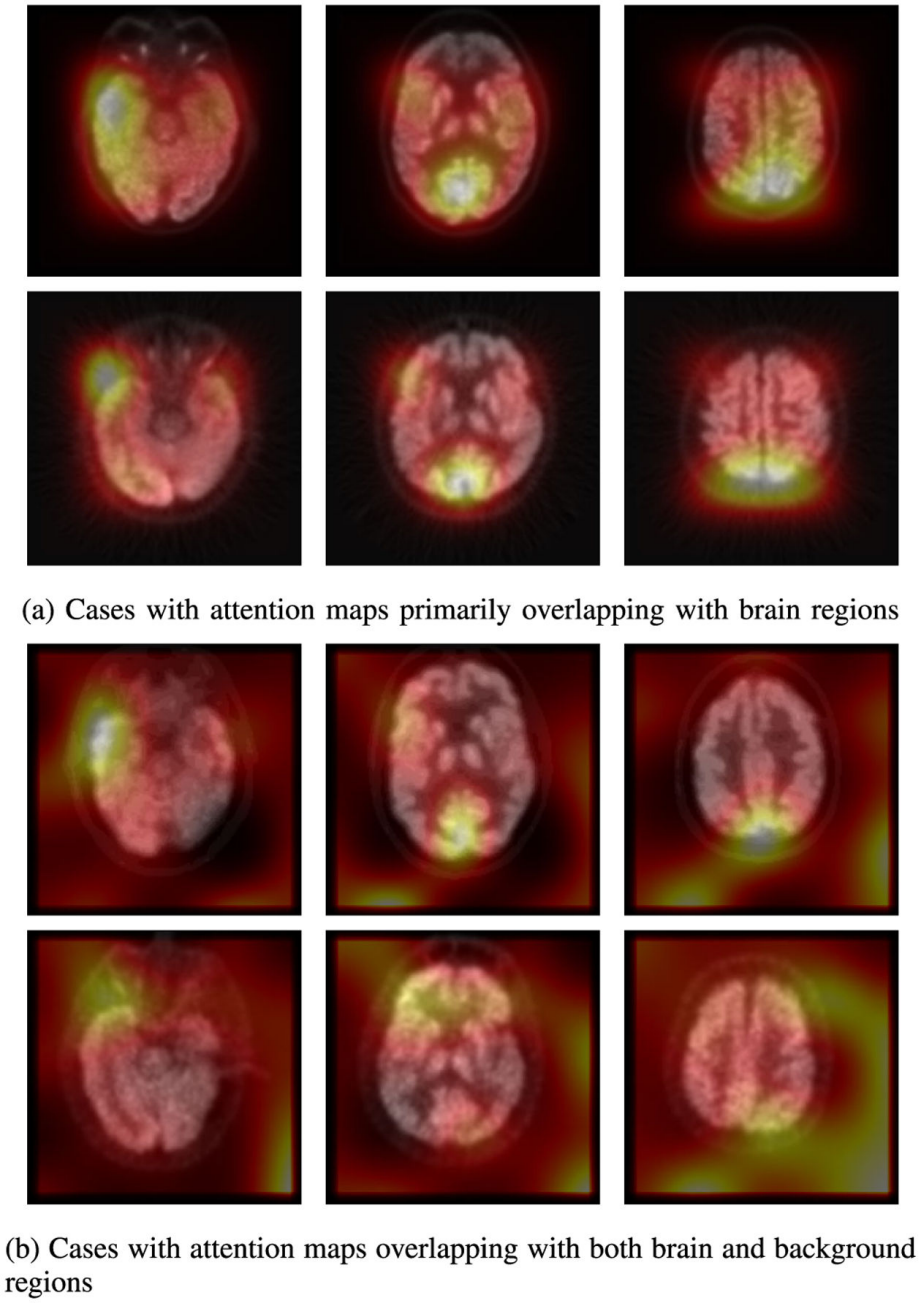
Attention maps on axial FDG-PET slices. Three axial slices on each row belong to the same PET scan.
